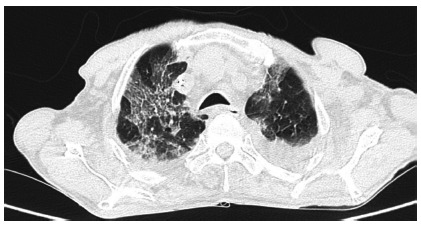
Chest CT scan taken on the 5th PO day
